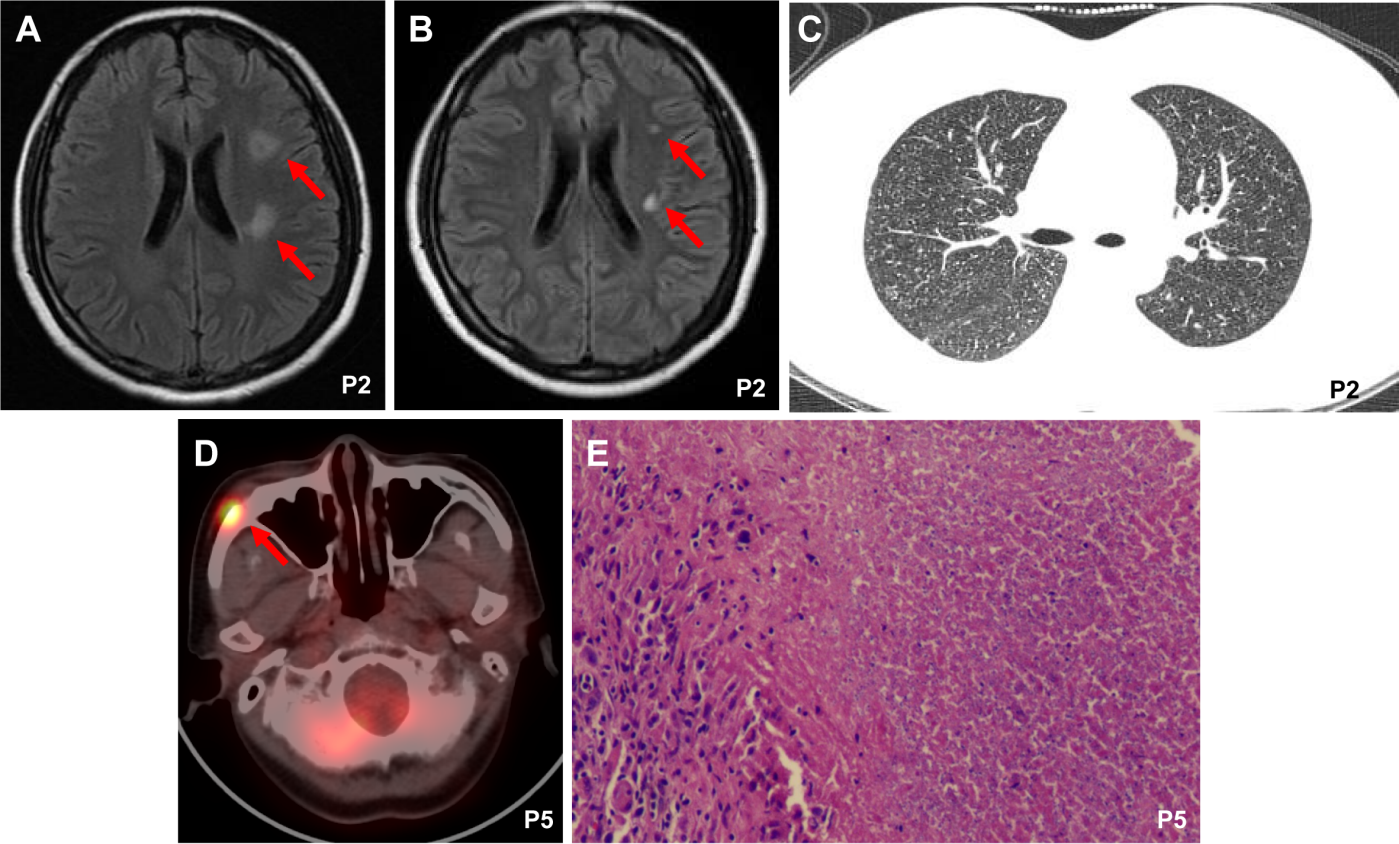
Imaging findings and tissue biopsy. (A) Cranial magnetic resonance imaging (MRI) showed focal FLAIR-weighted signal hyperintensity in patient 2 after 2 months of CAR19/22 T-cell cocktail therapy. (B) The MRI image of patient 2 after anti-TB therapy for one month. (C) Chest computerized tomography (CT) images showed disseminated miliary nodules in bilateral lung fields in patient 2 after 2 months of CAR19/22 T-cell cocktail therapy. (D) Positron emission tomography/computed tomography (PET/CT) scanning of patient 5 after 11 months of CAR19/22 T-cell cocktail therapy. (E) Pathology of the subcutaneous nodules on the right malar region in patient 5 revealed necrotizing granuloma. P, Patient.
